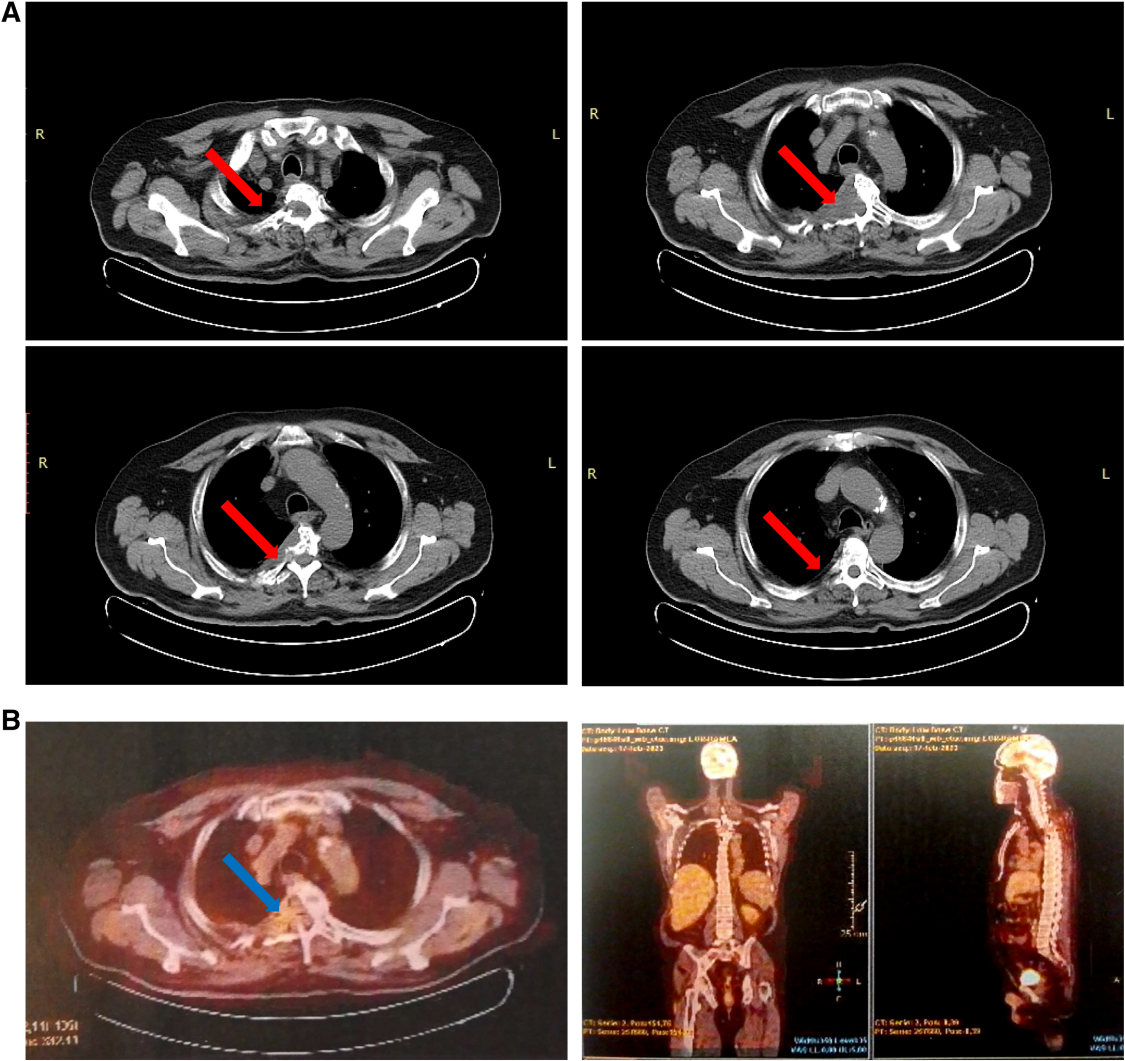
Imaging re‐evaluation. (A) CT and (B) FDG‐PET scan imaging showing a significant size reduction of pulmonary plasmacytoma (red arrows) with minimal residual uptake of radioactive marker (blue arrow).
